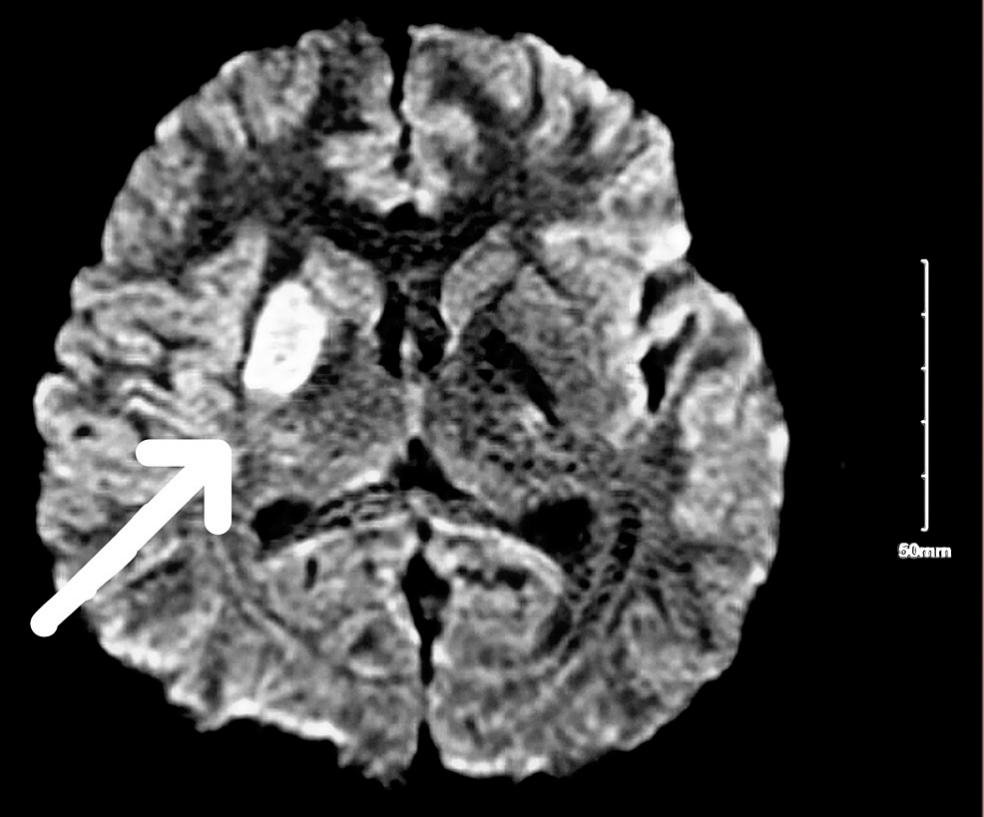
Magnetic resonance imaging of the brain showing acute right caudate nucleus and putamen infarcts with suggestion of faint petechial hemorrhage with mild mass effect and partial effacement of the frontal horn of the right lateral ventricle (white arrow). mm - millimeter
